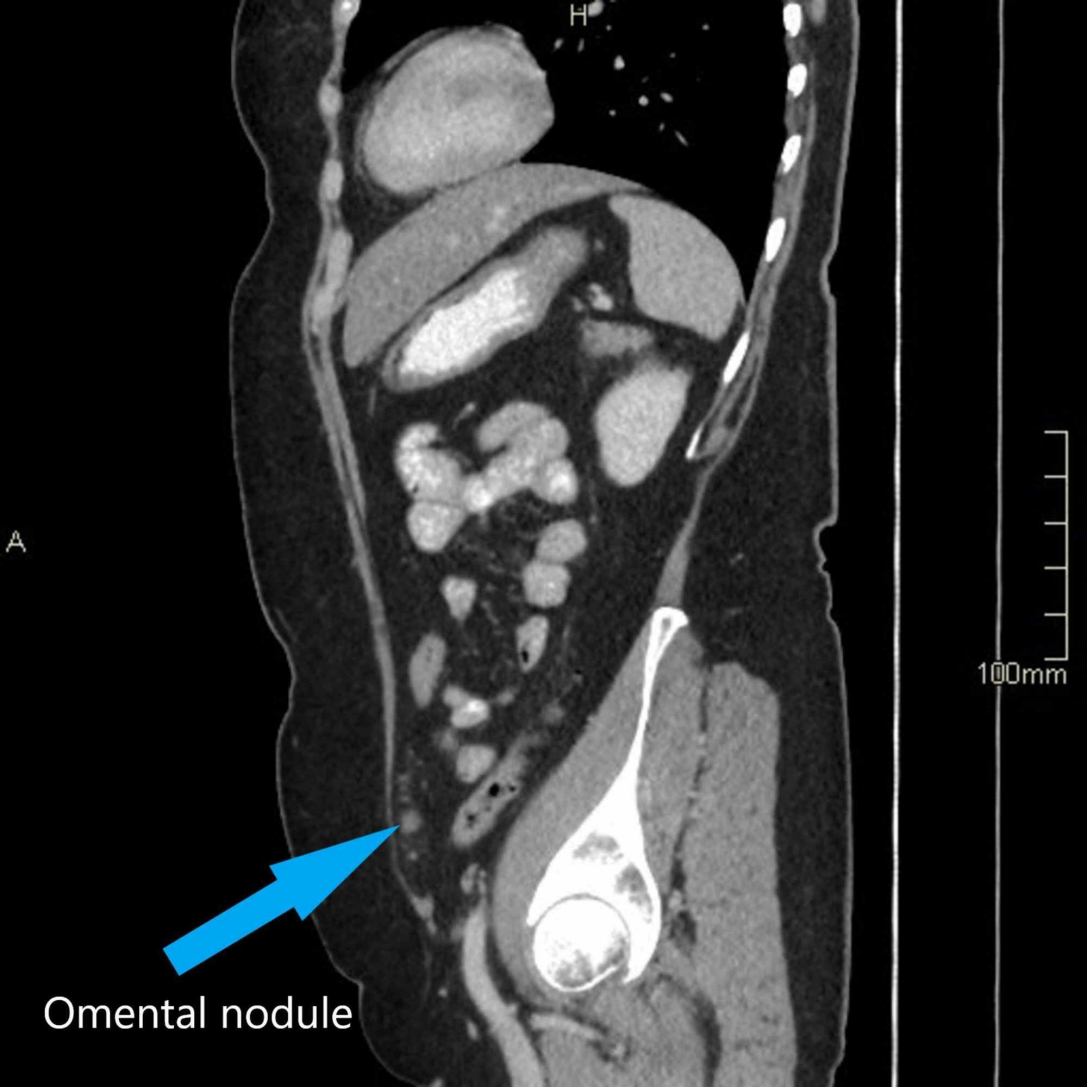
Sagittal view from CT scan
